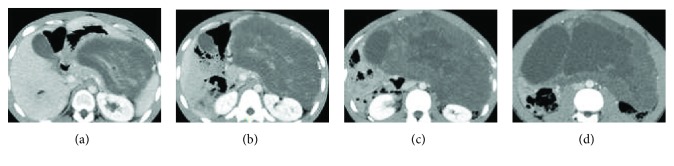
(a–d) CT scan with intravenous contrast (sagittal view): the tumor seemed to be divided into two parts. The right part was like a single cystic lesion; the left part contained a solid component.
